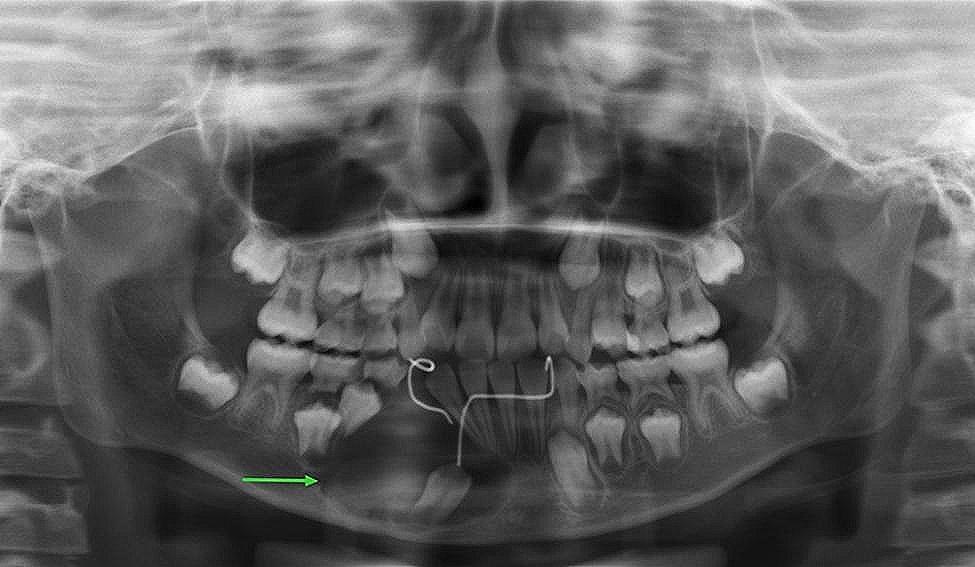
Preoperative panoramic radiography
